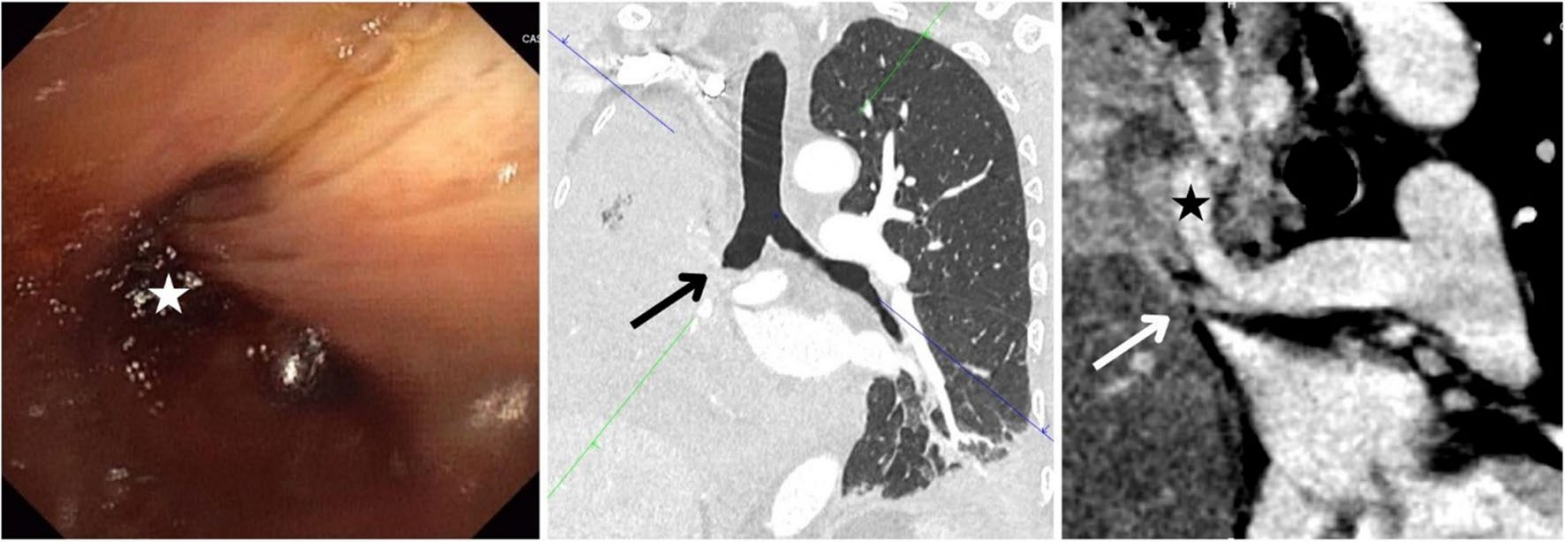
Bronchoscopy and computed tomography (CT) scan showing the hilar torsion. Bronchoscopy shows impenetrable obstruction of the right main bronchus (white star) with active bleeding (appearance of old blood). CT scan shows a sudden obstruction of the right main bronchus (black arrow). The CT scan shows a rotated artery (white arrow), the inferior pulmonary artery (black star) remains patent in the upper position, and the middle and superior pulmonary arteries are narrowed and beside the plane of this image
